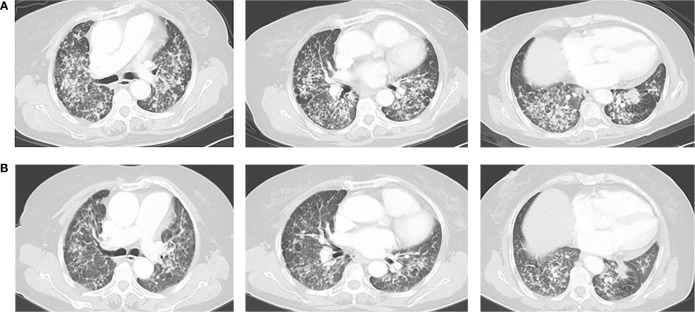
(A) Chest CT on October 25, 2018. (B) Chest CT on December 21, 2018. The response was partial response (PR).
